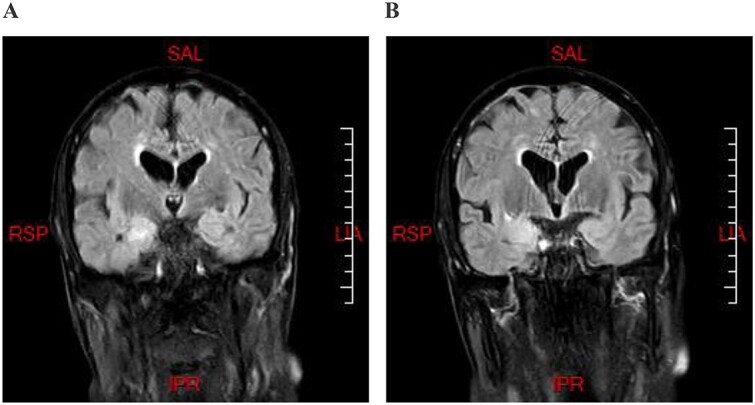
Axial brain MRI T2 FLAIR imaging demonstrating bilateral hyperintensity in the medial temporal lobe prominent on the right.
